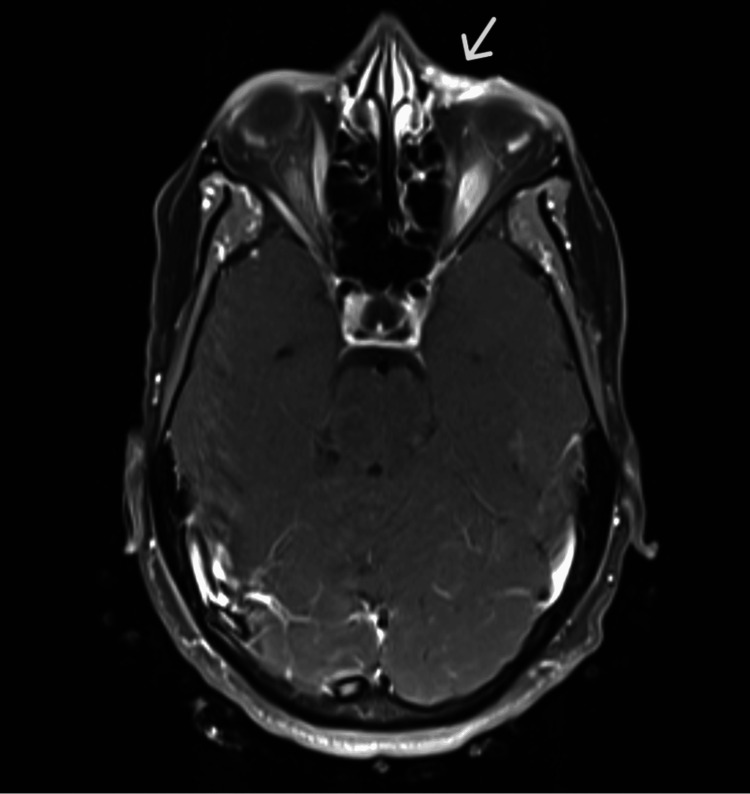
An MRI image showing enhancement in the left lower eyelid (with IV contrast)
